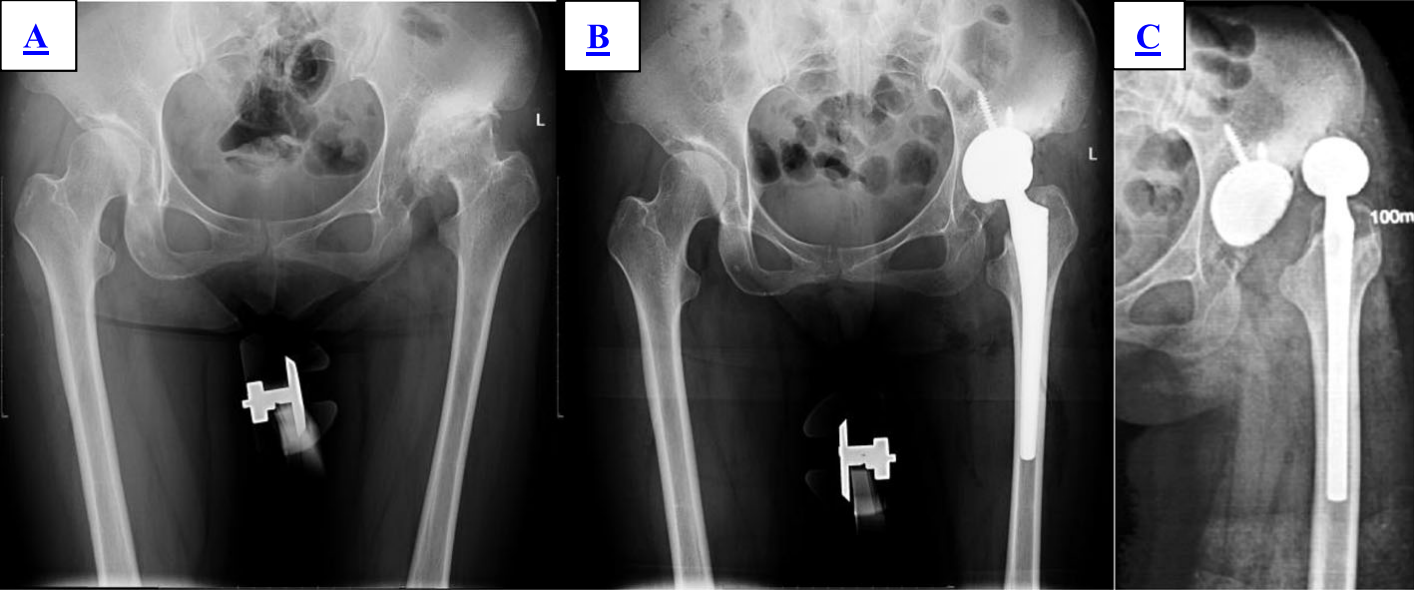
Female, 38 years old. a Preoperative X-ray showed left Crowe II DDH. b Postoperative X-ray. c Anterior dislocation in the fourth day postoperatively
